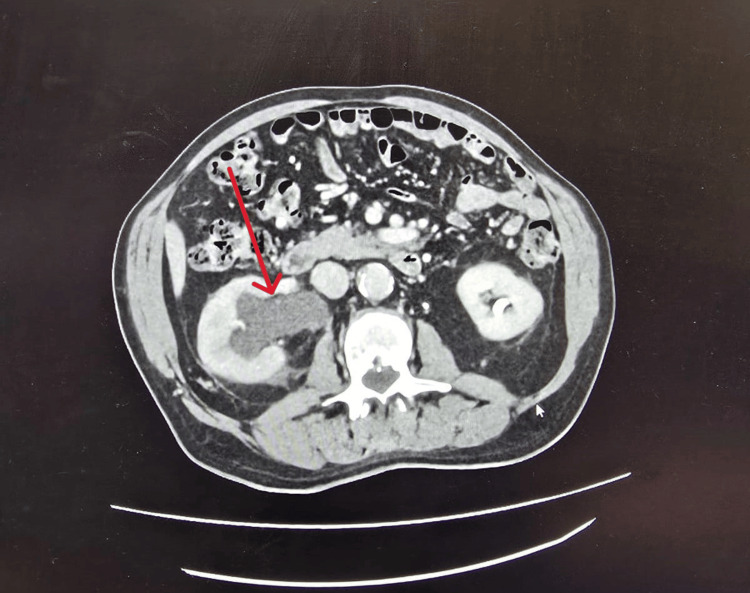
CT Urogram transverse section . CT Urogram transverse section showing right hydroureter (Red Arrow) leading into right hydronephrosis.
